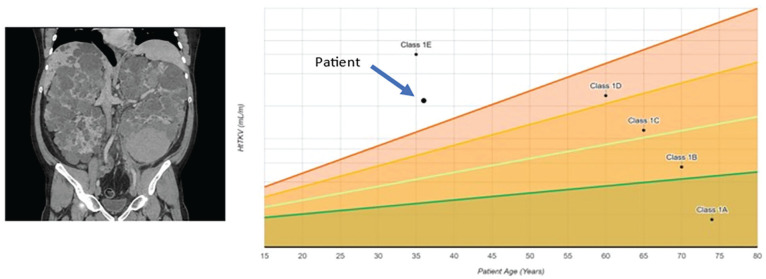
Magnetic resonance imaging and Mayo clinic risk stratification of clinical vignette.
